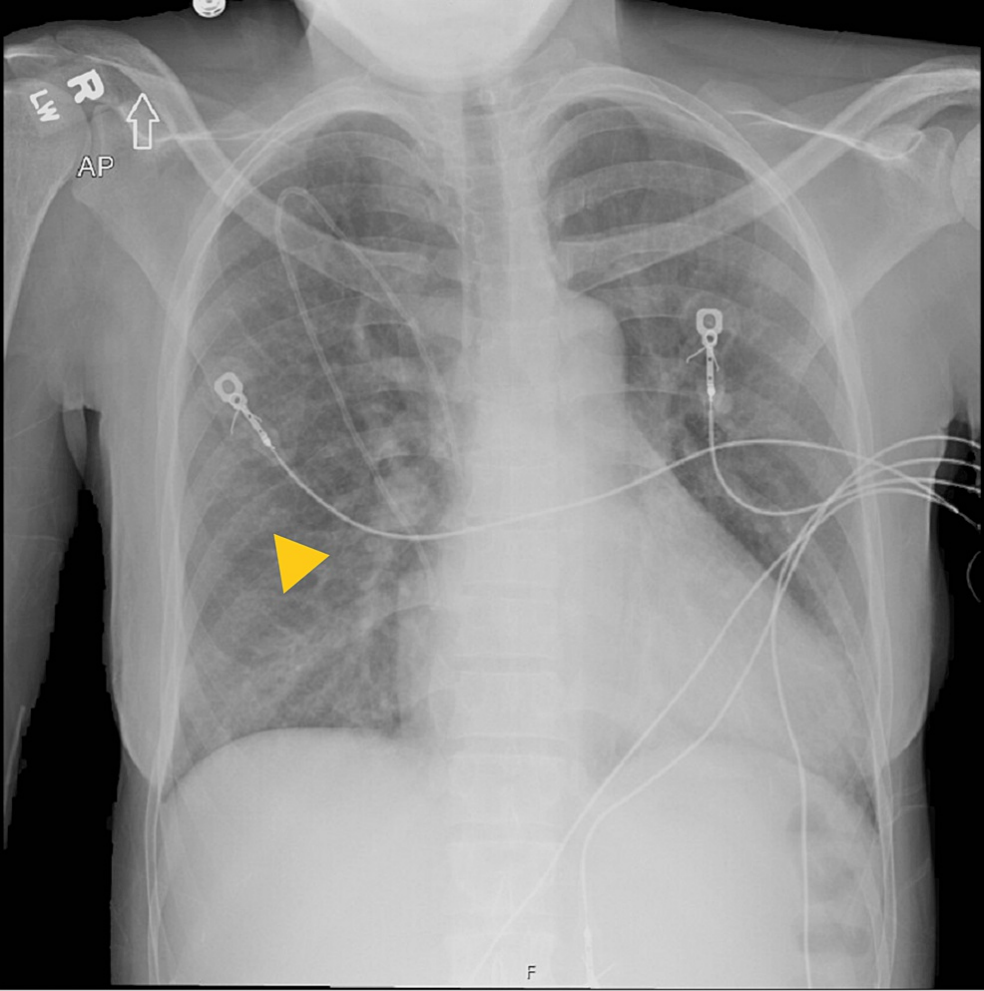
Chest X-ray suggestive of interstitial edema vs viral or atypical pneumonia
